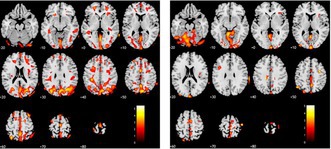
FIGURE 2 FDG‐PET hypometabolism patterns in a GBA1‐associated Parkinson's disease patient (59‐year‐old male) and an unaffected GBA1 carrier (56‐year‐old female), obtained using single‐subject SPM analysis versus 125 age‐corrected healthy controls (*p* < 0.05).
